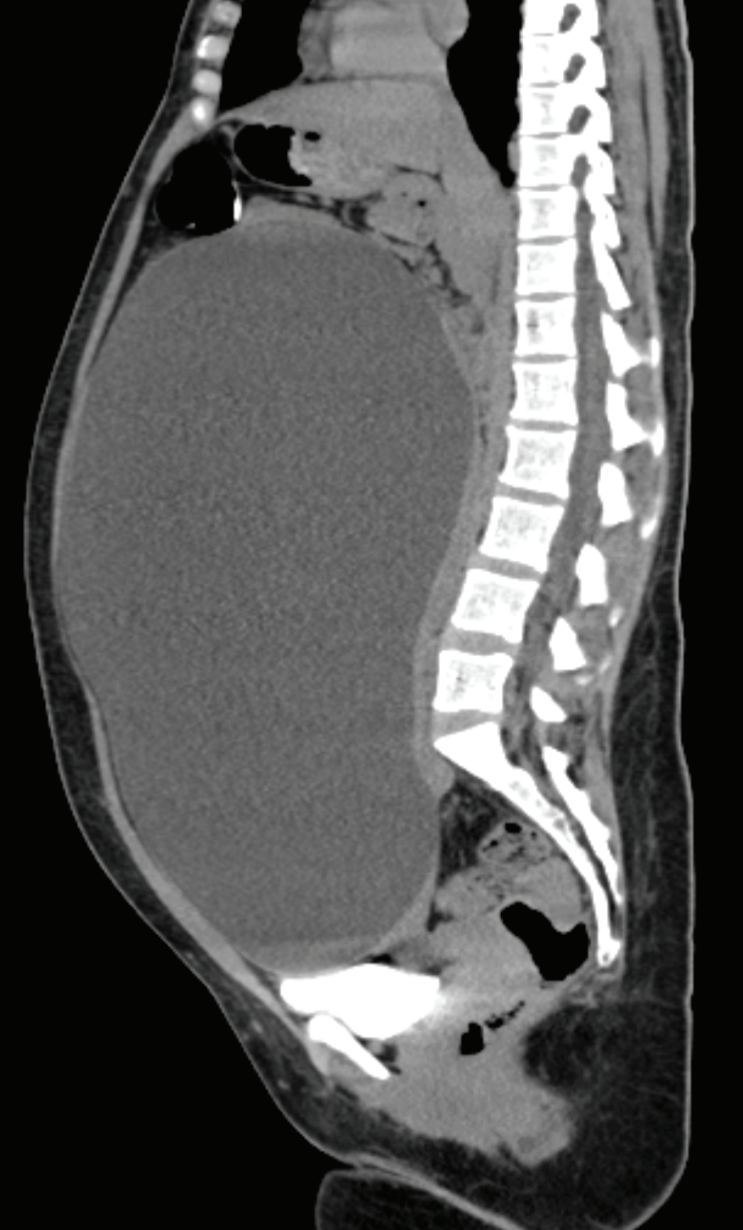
CT scan of cystic abdominopelvic mass.
